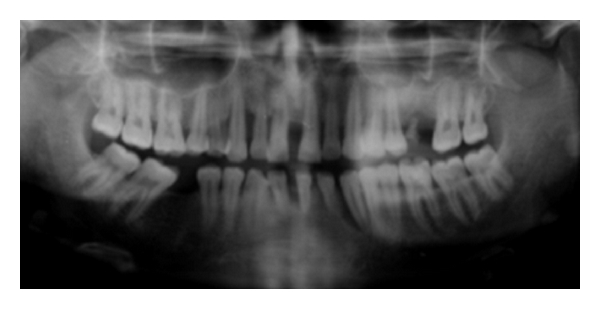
Orthopantomogram showing generalized bone loss with advanced vertical defect in 13 and 33 regions.
